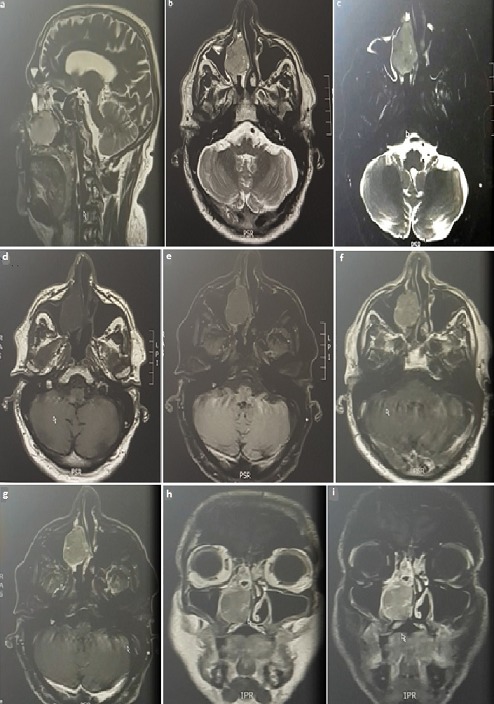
Nasosinusal MRI showing the tissue mass measuring 4 × 2.8 × 3.5cm with ethmoidal sinus fluid retention without intracranial or orbital extension (on sequence: (A) sagittal T2; (B) axial T2 TSE Fast; (C) axial T2 DIXON Fat; (D) axial T1 TSE dixon in; (E) axial T1 TSE dixon W; (F) axial T1 TSE dixon Gadolinium in; (G) axial T1 TSE dixon Gadolinium W; (H) coronal T1 Gadolinium; (I) coronal T1 Gadolinium W)
